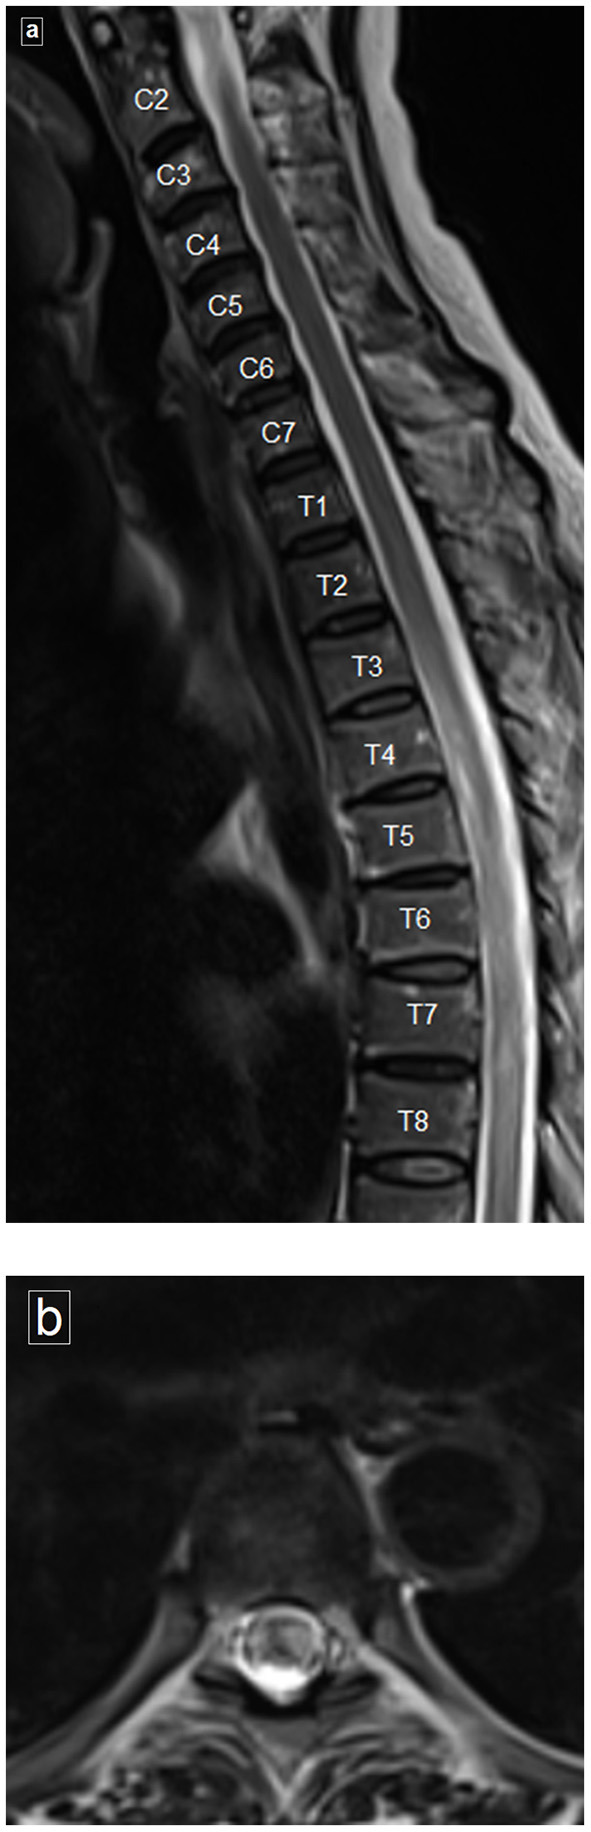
Magnetic resonance imaging (MRI) of spine. (a) Sagittal T2-weighted image of the cervical and dorsal spine reveals poorly delineated long segment of hyperintense signal, clearly evident from T4 to T8. (b) Axial T2-weighted image showing the hyperintense signal involving more than two thirds of the cross-sectional area of the cord.
